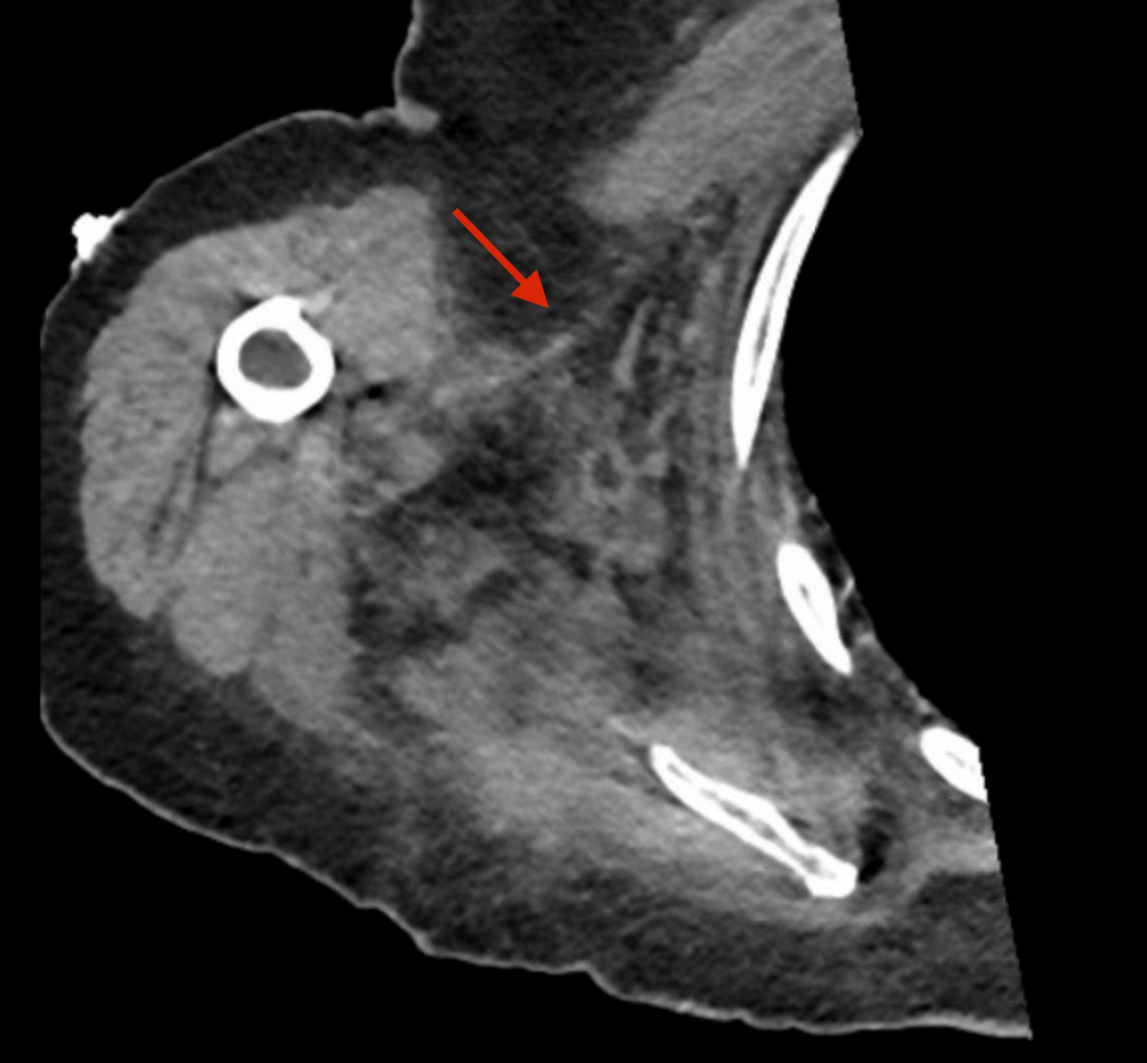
CT scan of the right shoulder and thorax showing right thoracic soft tissue edema (arrow).
